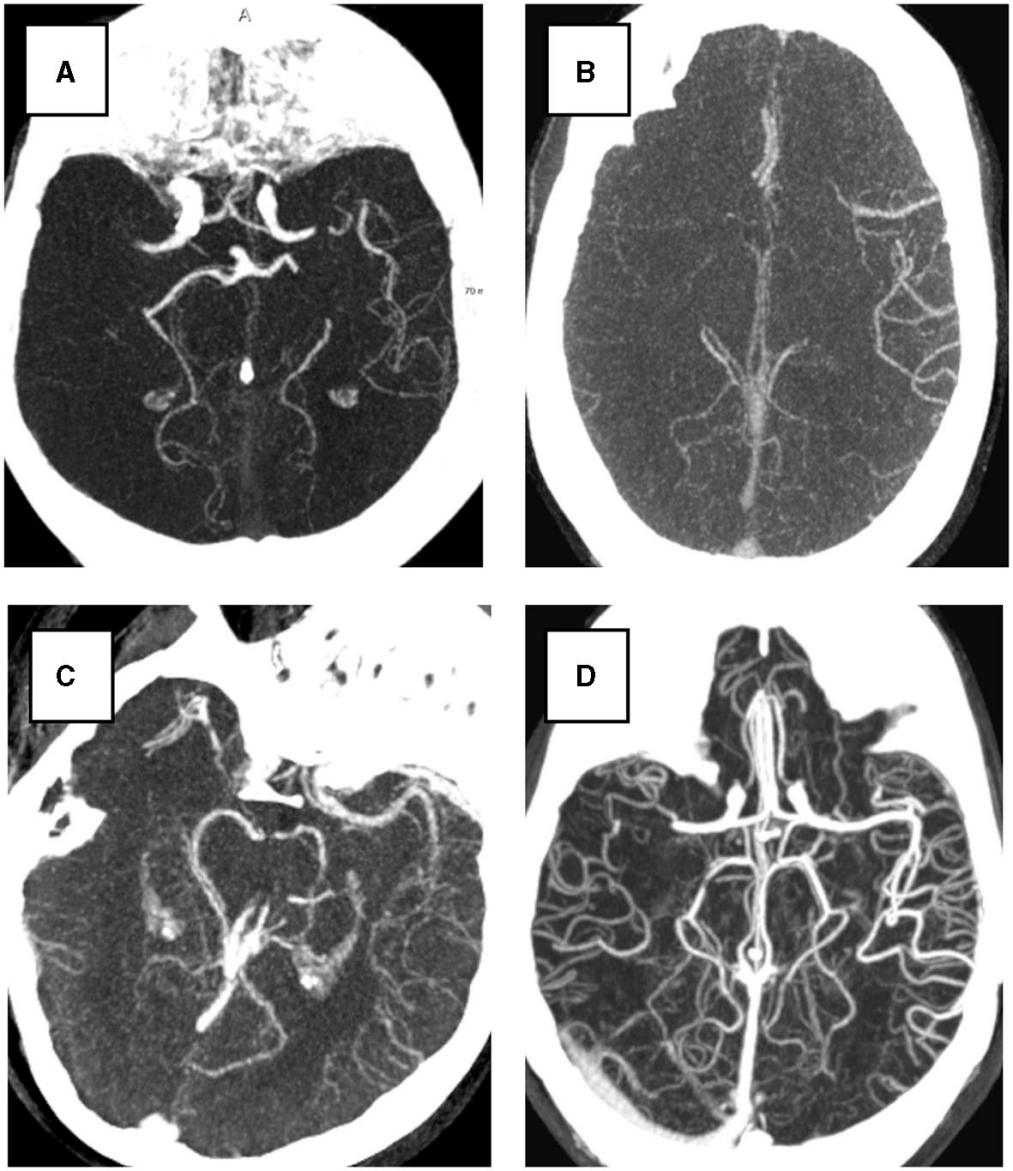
Examples of four collateral score in Maximum Intensity Projection (MIP) from 3D CTA image. All subjects have a right hemisphere occlusion. (A) Collateral score 0; (B) Collateral score 1; (C) Collateral score 2; (D) collateral score 3.
